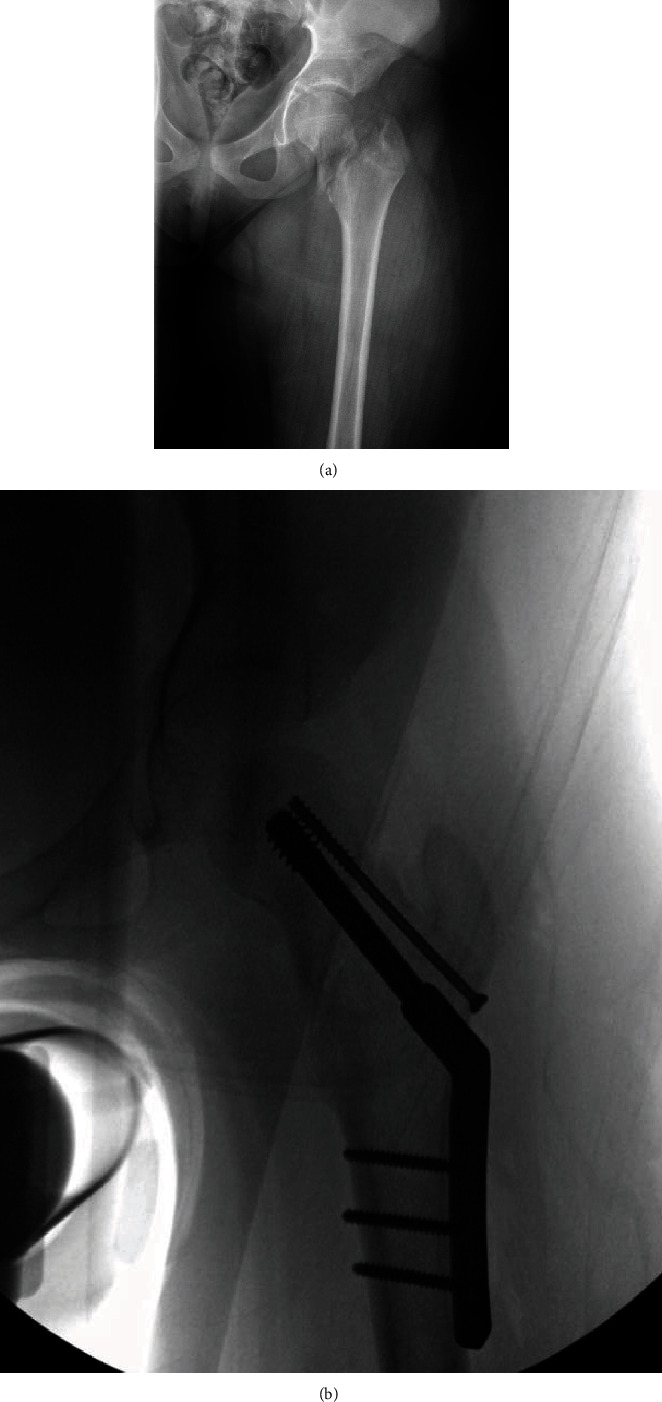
(a) X-ray image of patient's injury; (b) open reduction and internal fixation RIF of intertrochanteric left femur fracture with dynamic hip screw.
